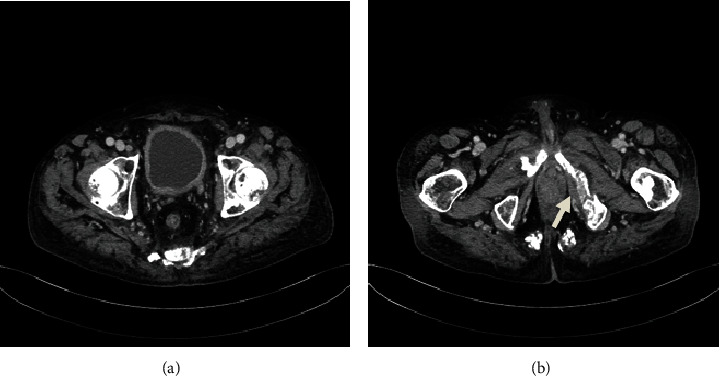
CT findings four weeks after starting pembrolizumab therapy revealed a significant reduction in the size of the primary lesion (a), as well as a decrease in osteolytic lesions at the bone metastatic sites with a shift towards osteoblastic change (b, arrow).
